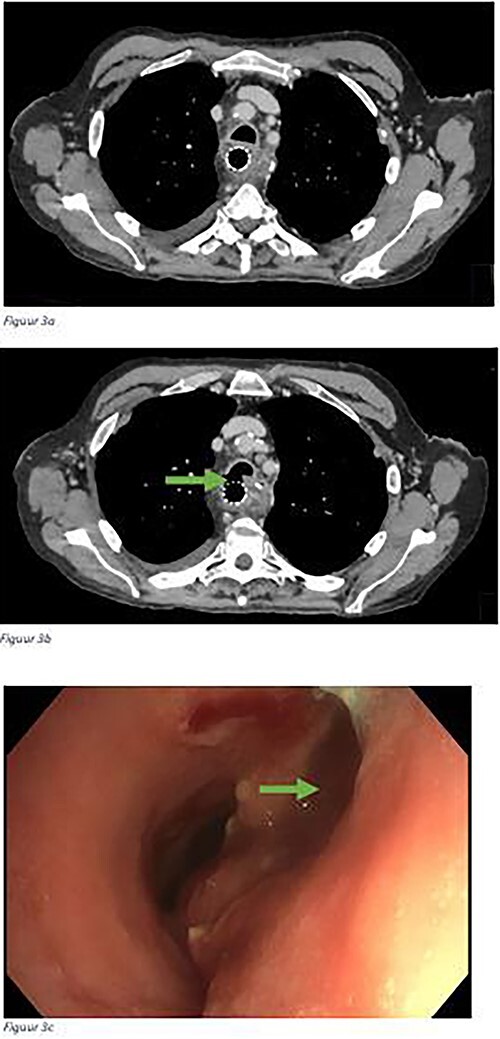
A CT-scan of a patient with an esophago-tracheobronchial fistula with a stent in the gastric conduit and patent airway in (a) and a visible defect in the trachea on (b) which was also observed during bronchoscopy (c). The arrow is pointing to the esophago-tracheobronchial fistula.
